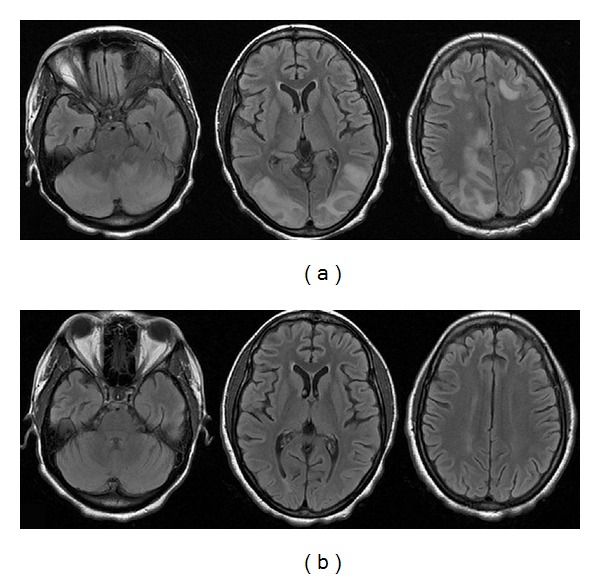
(a) Axial MRI scan (FLAIR sequence) displays multiple bilateral hyperintense lesions affecting the white matter of cerebellar hemispheres and of frontal, occipital, and parietal lobes. (b) At followup (one week later) the same sequence shows a complete resolution of all findings.
